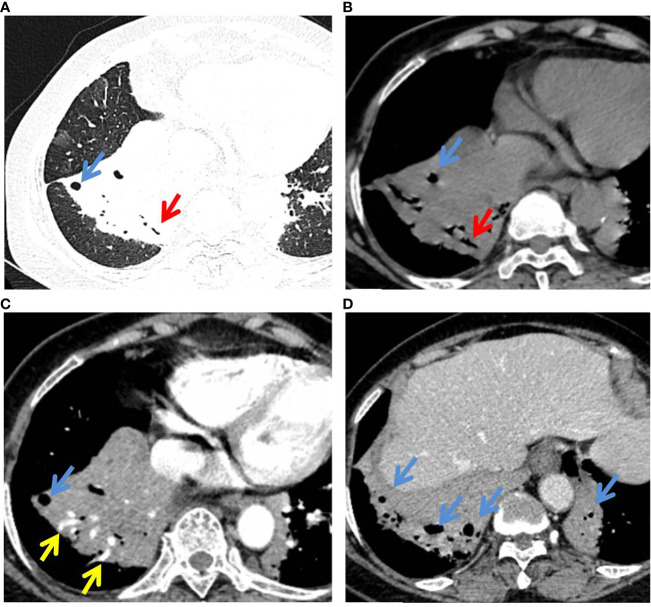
P-MALT lymphoma in a 66-year-old with a cough for 14 days. (A) Axial CT image of the lung window indicated multiple consolidations with air bronchogram (red arrow) and cystic bronchiectasis (blue arrow) in the left lower lobe and right lower lobe. (B–D) Mediastinum-window in the plain scan, arterial phase, and venous phase showed air bronchogram (red arrow), cystic bronchiectasis (blue arrow), and angiogram sign (yellow arrow) within the lesions. CT, computed tomography; C-MALT, consolidation pattern of pulmonary mucosa-associated lymphoid tissue.
